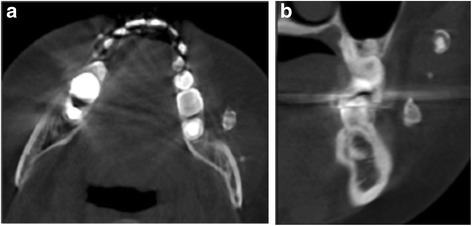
Preoperative computed tomography imaging shows multiple radiopaque masses inside the left masseter muscle. a Axial. b Coronal
